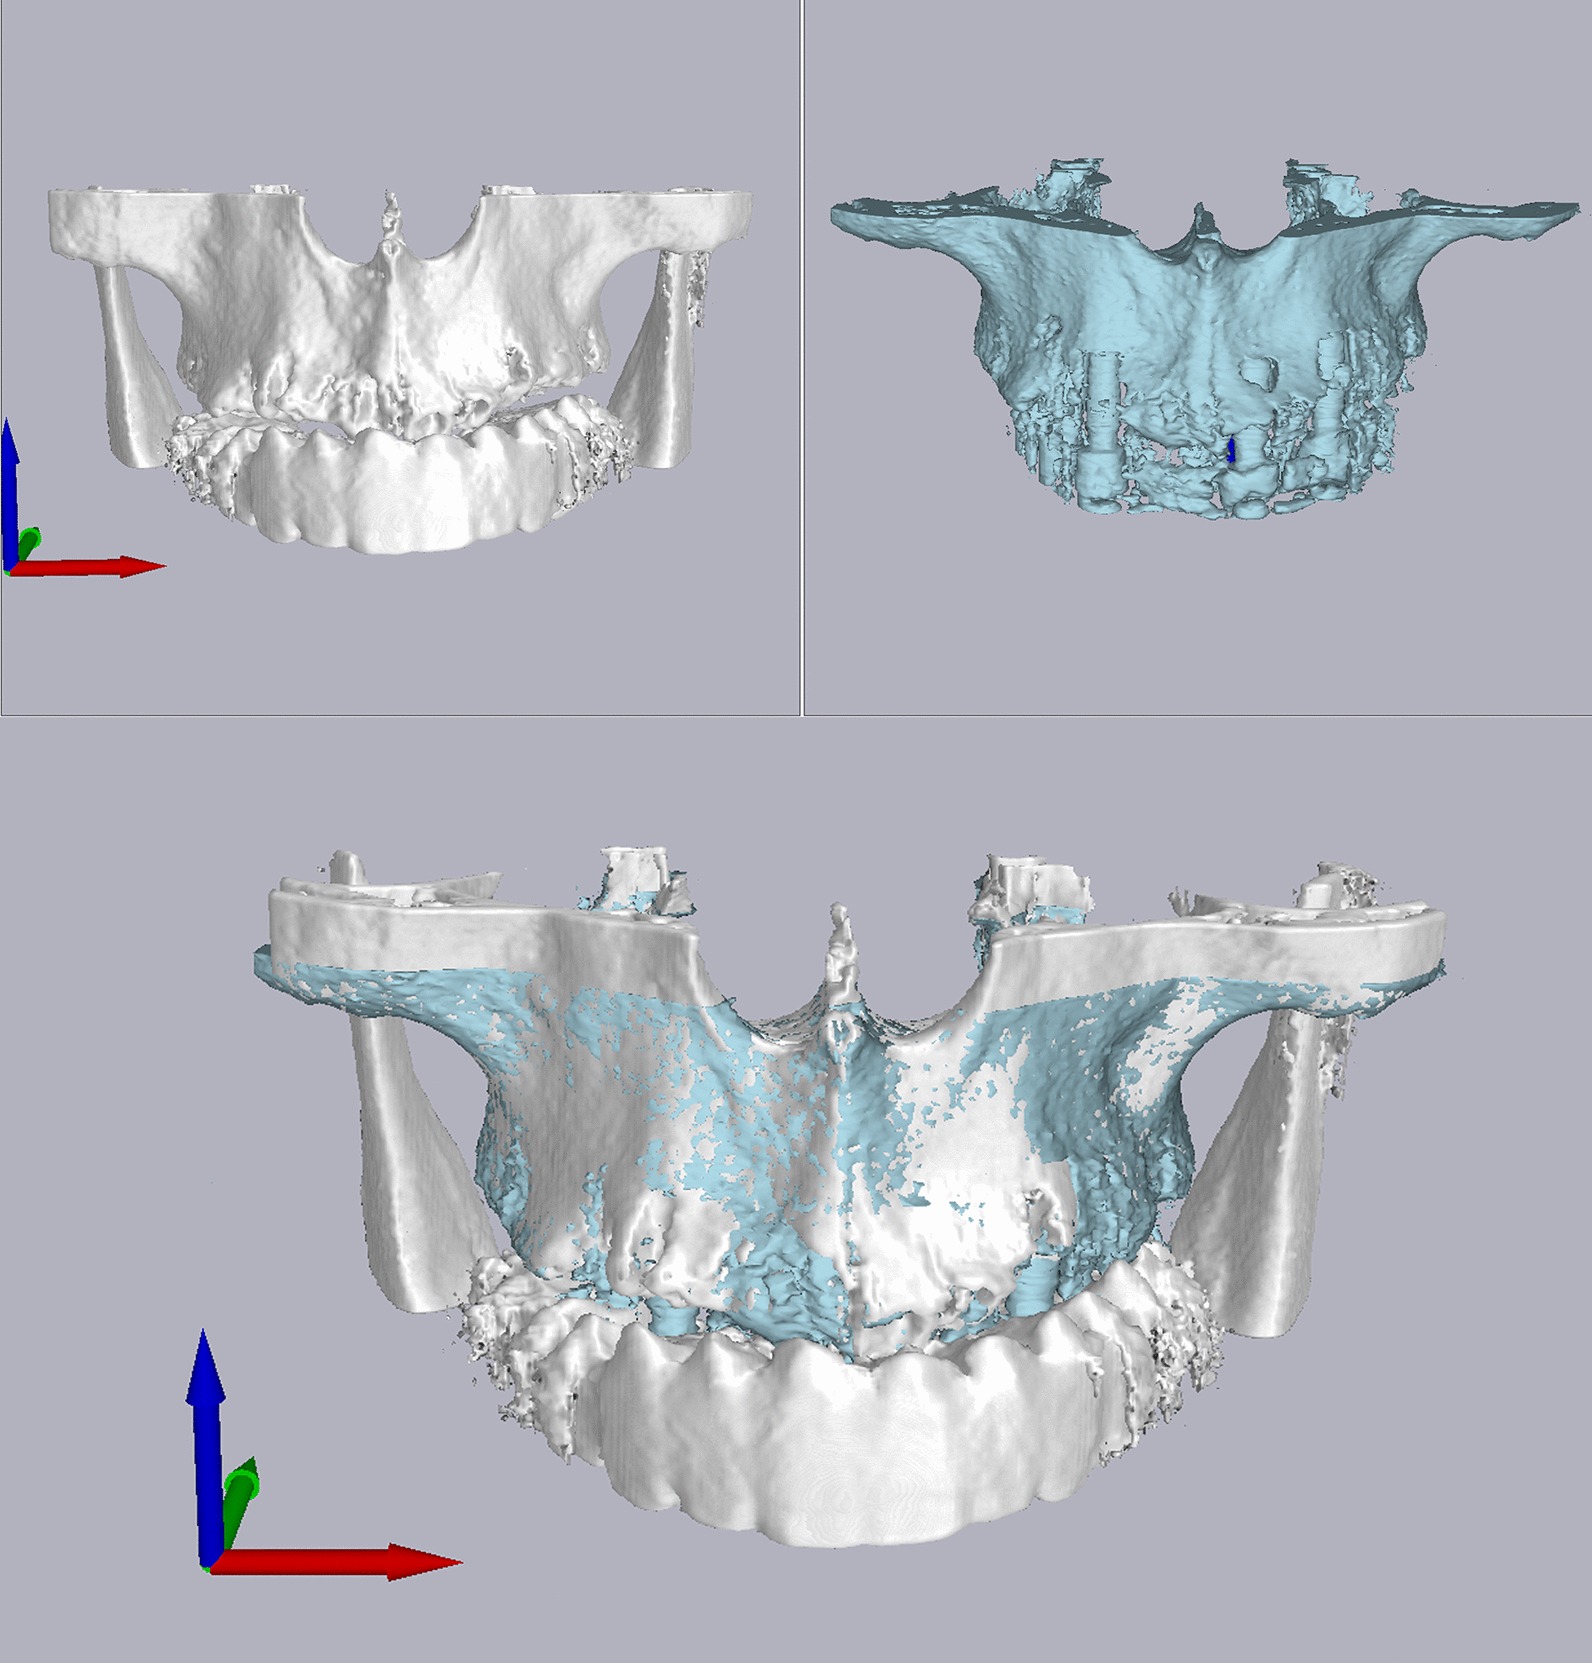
The superimposition of the pre- and post-operative data reconstructed from the CBCT scans
